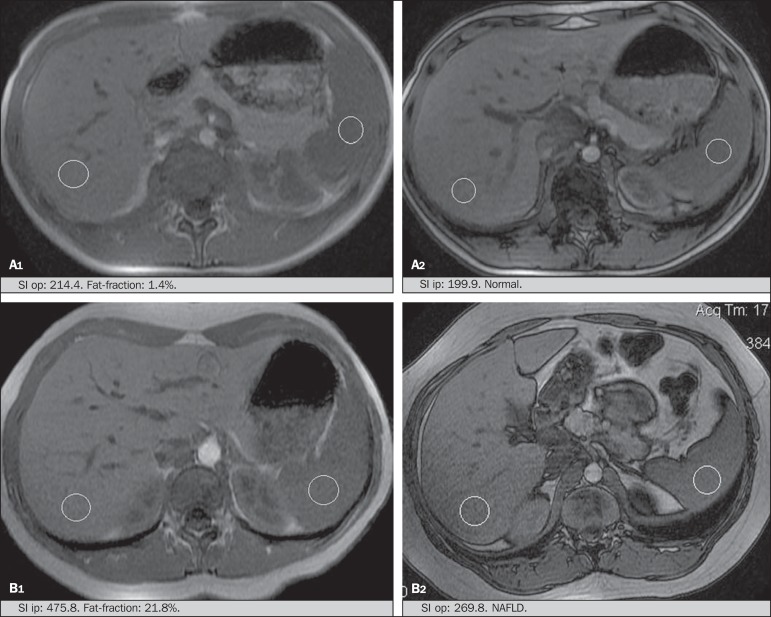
A: MRI in-phase , out-of-phase and chemical shift images
